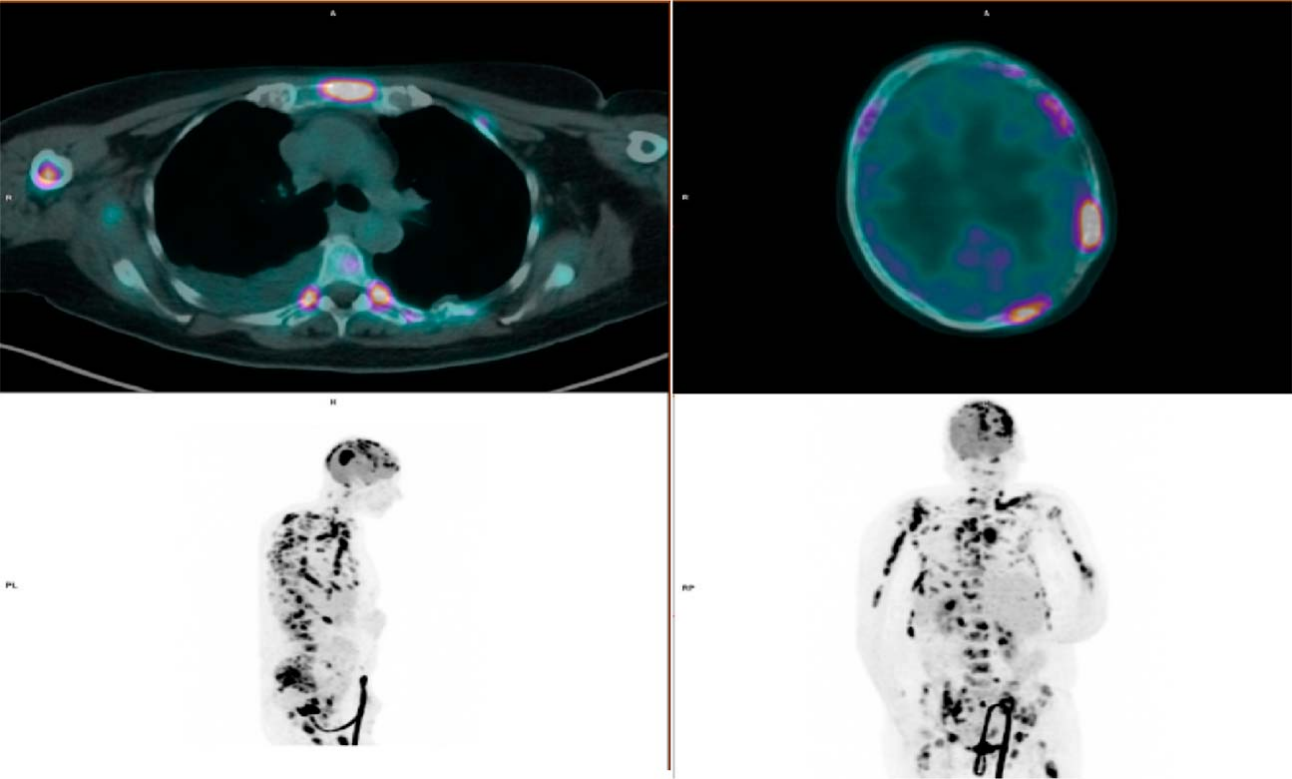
PET-CT scan: multiple osteolytic bone lesions with high avidity for 18F-FDG and low metabolism in the left cervical lymph node and right lower lobe.
